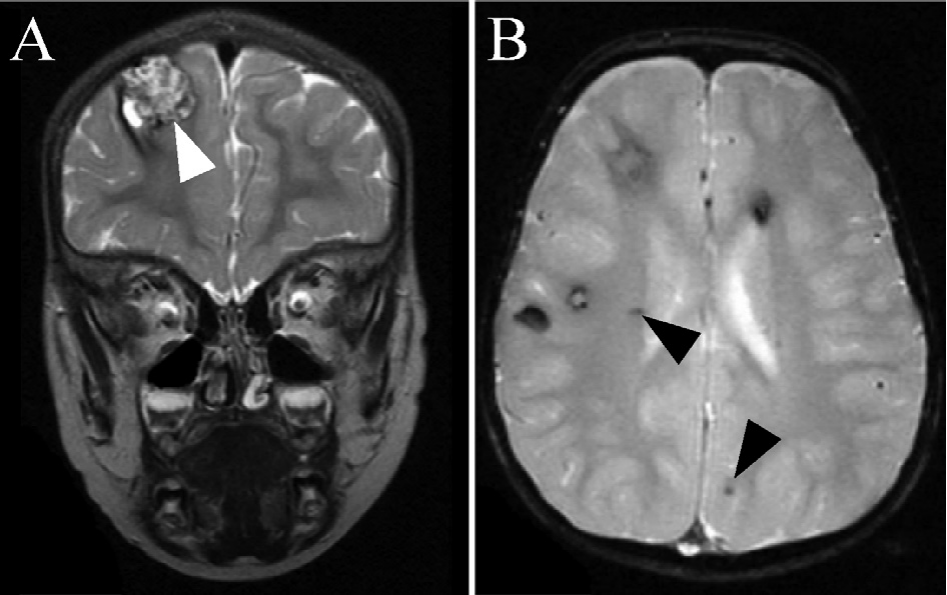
Characteristic MRI findings in CCM. MRI of a 1-year-old *CCM1* mutation carrier showing coronal (A) T2 and (B) axial T2*w sequences. (A) There is a huge mulberry-like cavernoma in the right frontal lobe (white arrowhead). Blood-degradation products of varying age cause a reticulated signal within the cavernoma. (B) T2*w imaging reveals multiple hypointense areas corresponding to hemosiderin depositions of several larger and dot-sized cavernomas (arrowheads).
